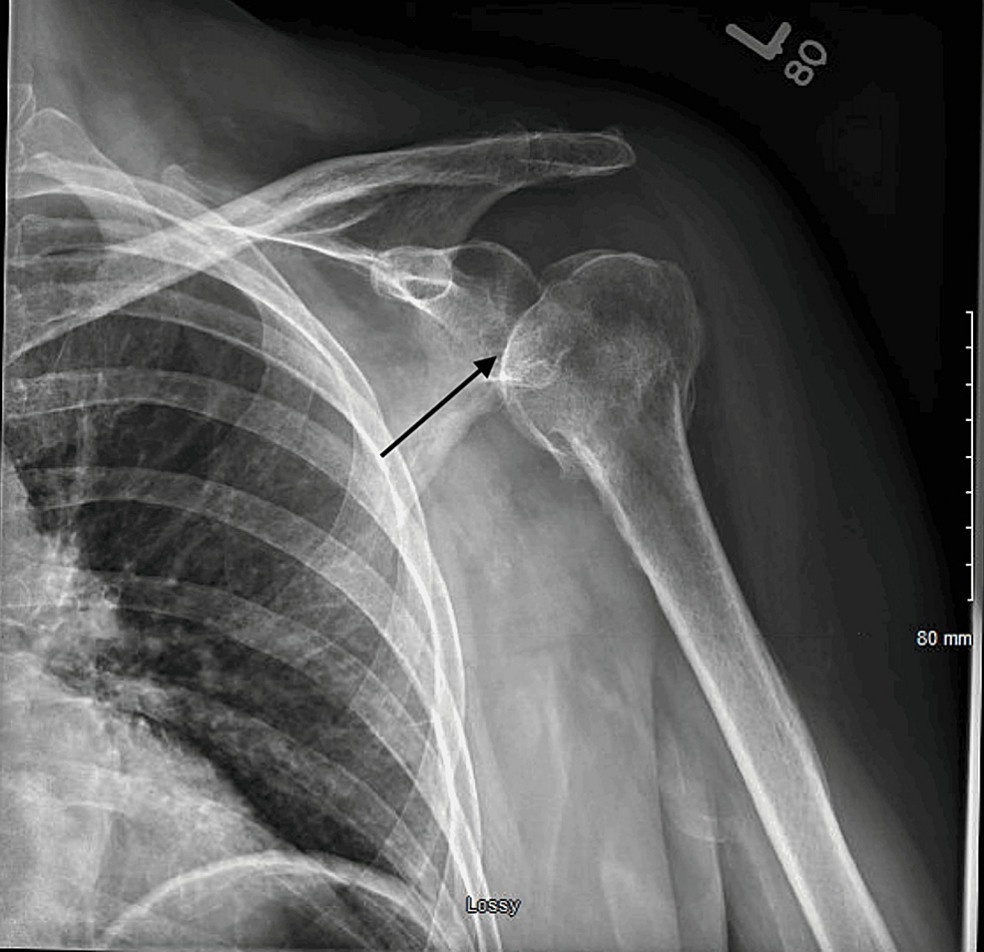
Shoulder x-ray showing a possible small effusion
